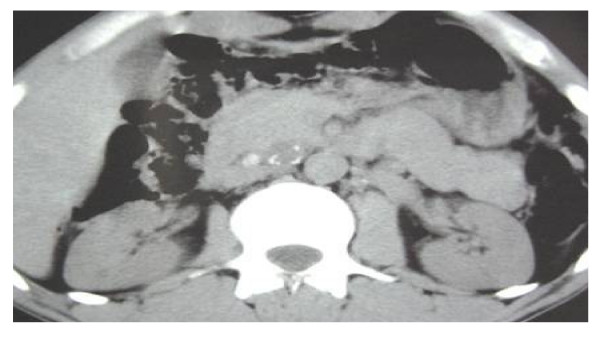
CT scan demonstrating several retroperitoneal masses, located in pre-aortic and inter aortocave, measuring between 2 and 5 cm, cystic aspect with some heterogenic focuses.
